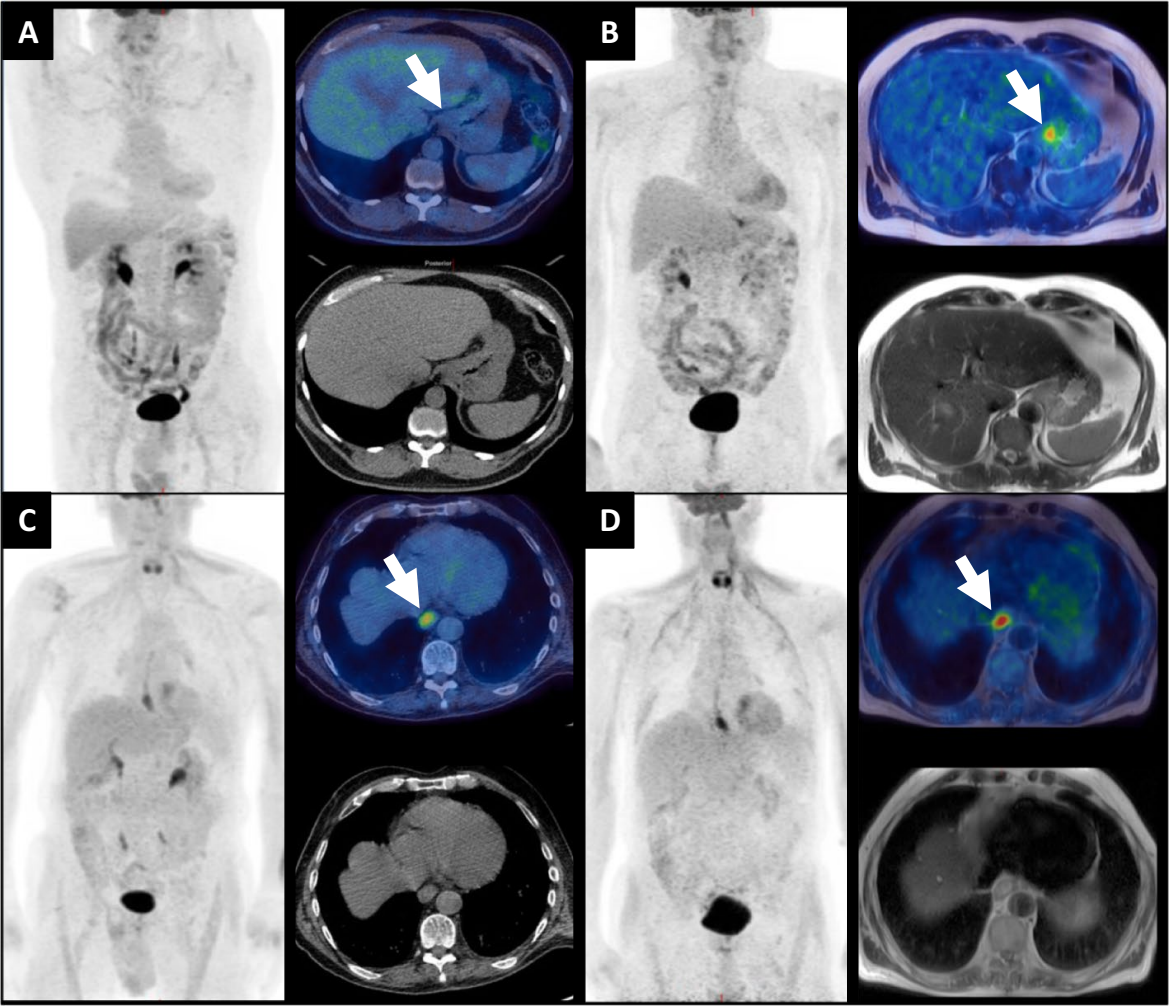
a, b A gastro-oesophageal adenocarcinoma, where uptake is noted on 18F-FDG PET/MRI (b) (highlighted by arrow), but lower corresponding uptake is noted on the 18F-FDG PET/CT (a). SUVmax was 9.4 on the 18F-FDG PET/MRI vs. 3.4 on the 18F-FDG PET/CT. c and d A lower oesophageal adenocarcinoma (highlighted by arrow), for which corresponding uptake is noted on both 18F-FDG PET/CT (c) and 18F-FDG PET/MRI (d). The SUVmax was 11.8 on the 18F-FDG PET/MRI vs. 7.2 on the 18F-FDG PET/CT
